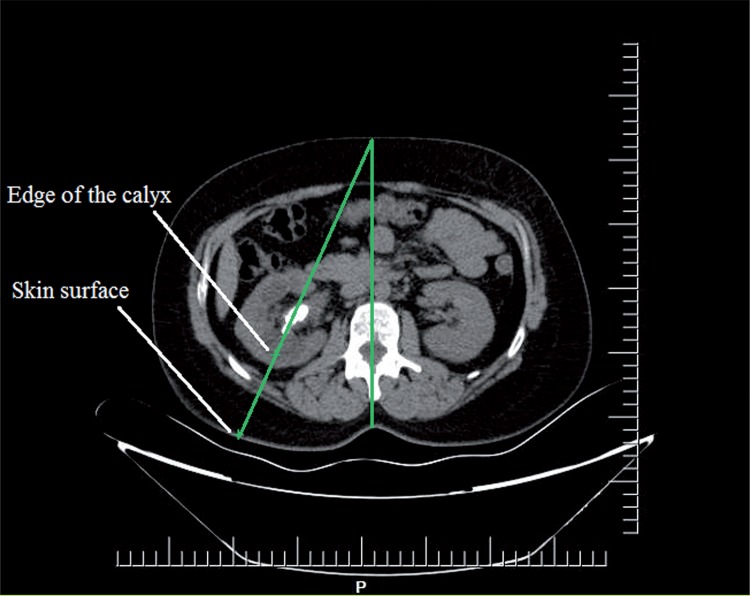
Example of an axial image from a CT scan, demonstrating the way 'Skin to calyx distance'calculated.
